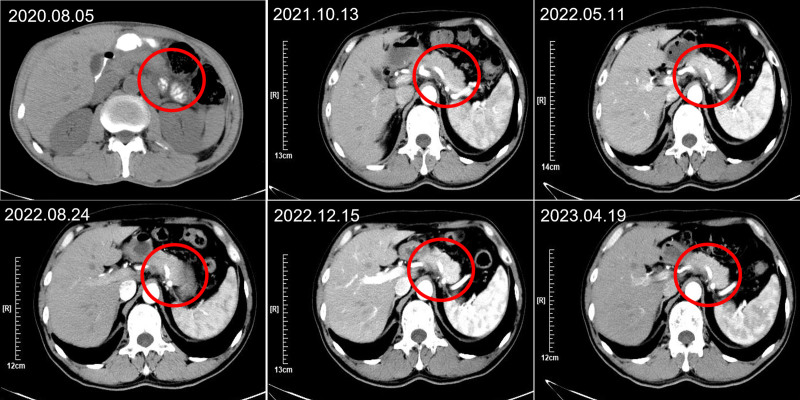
The representative CT images of case 2 after treatment. CT = computed tomography.
